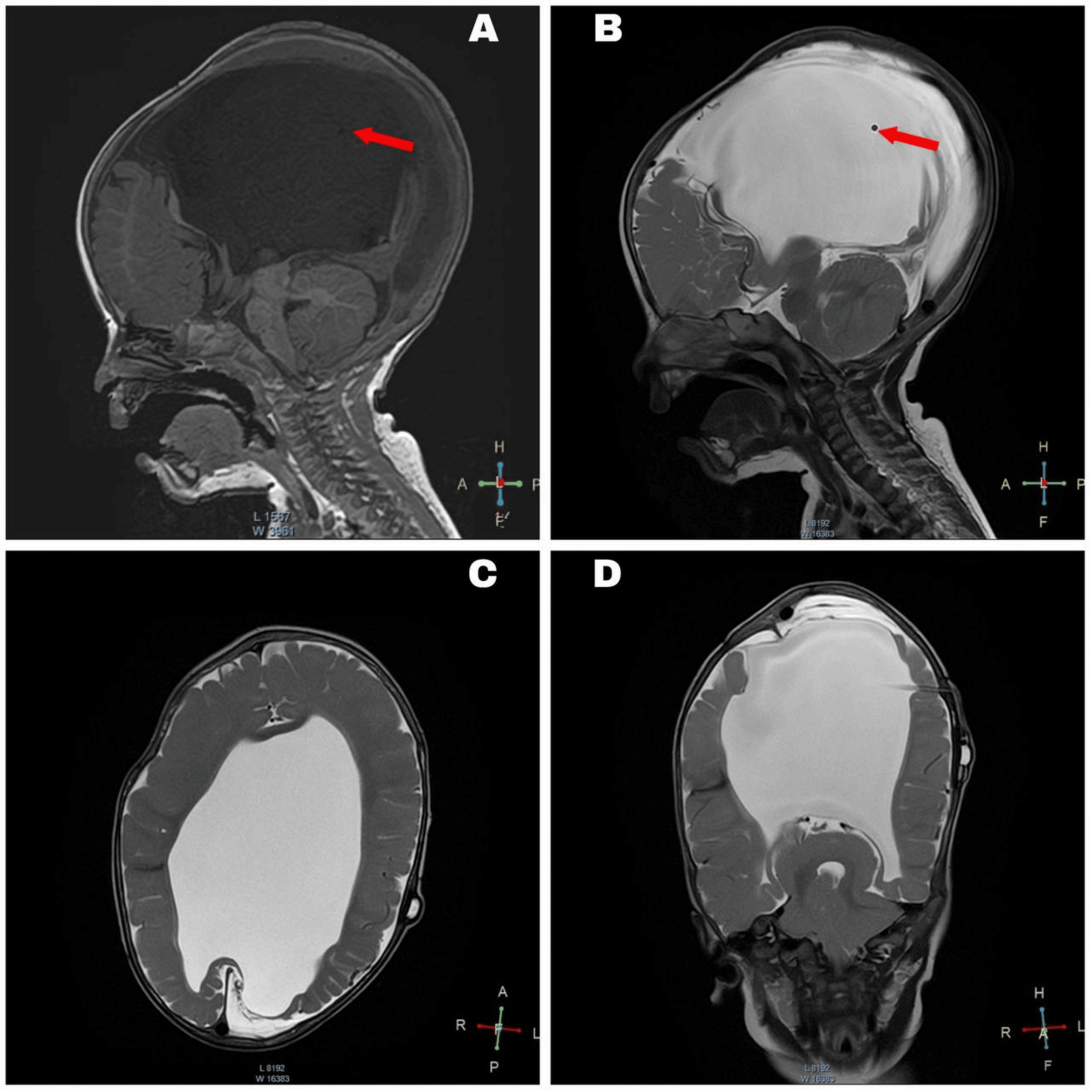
Follow-up magnetic resonance imaging of the brain at 12 months A-B: Sagittal view; red arrows indicate ventriculoatrial shunt catheter placement. C: Axial view. D: Coronal view; severe and asymmetric supratentorial hydrocephalus.
